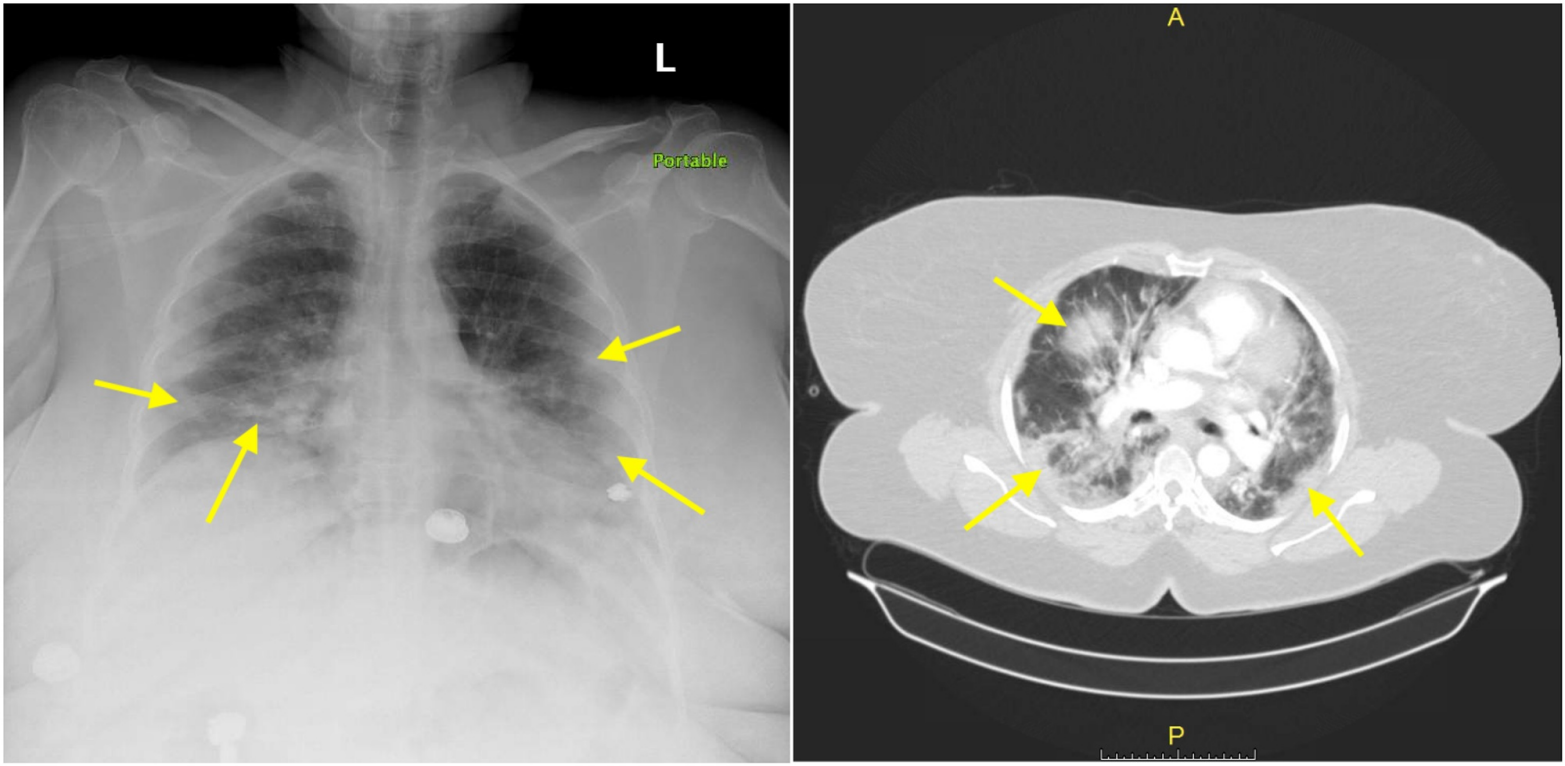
Chest X-ray (on left) and CT angiogram of the chest (on right) showing bilateral patchy pulmonary infiltrates (yellow arrows) concerning for multilobar pneumonia.
